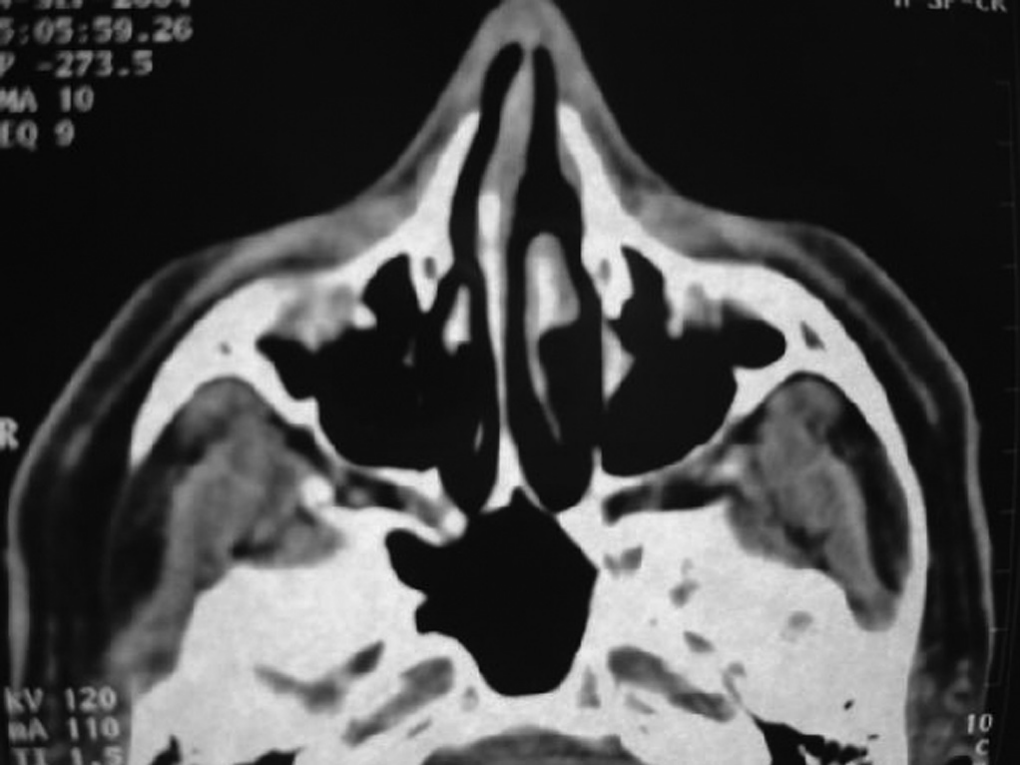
Postoperative CT: complete removal of the lesion.
